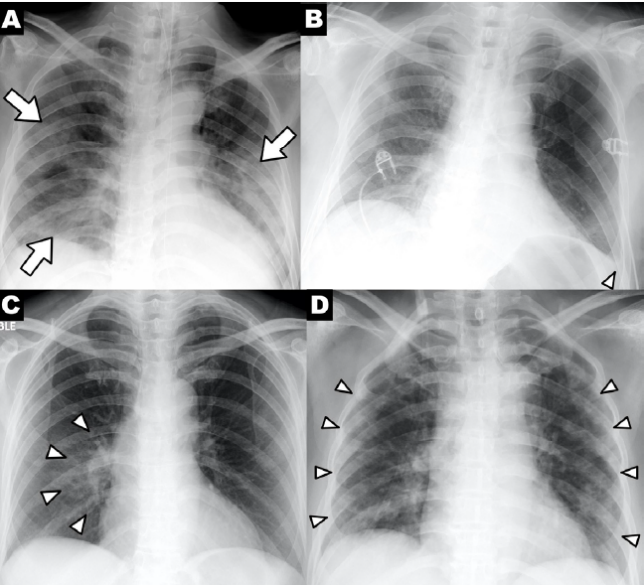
Chest x-ray findings in a patient with coronavirus disease. (A) Patchy consolidations, (B) pleural effusion, (C) perihilar distribution, (D) peripheral distribution [18].
